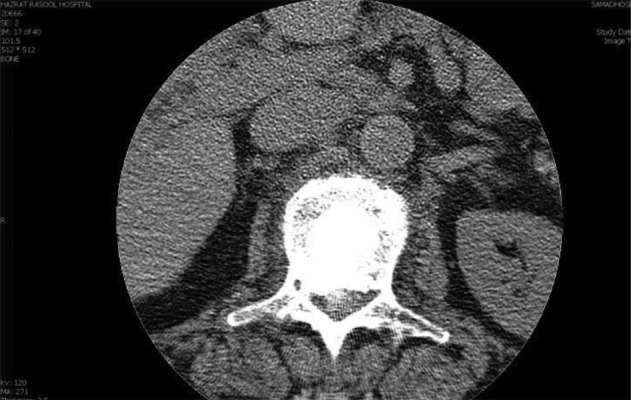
CT Scan After Kyphoplasty (Vertebra is Filled With Cement)
